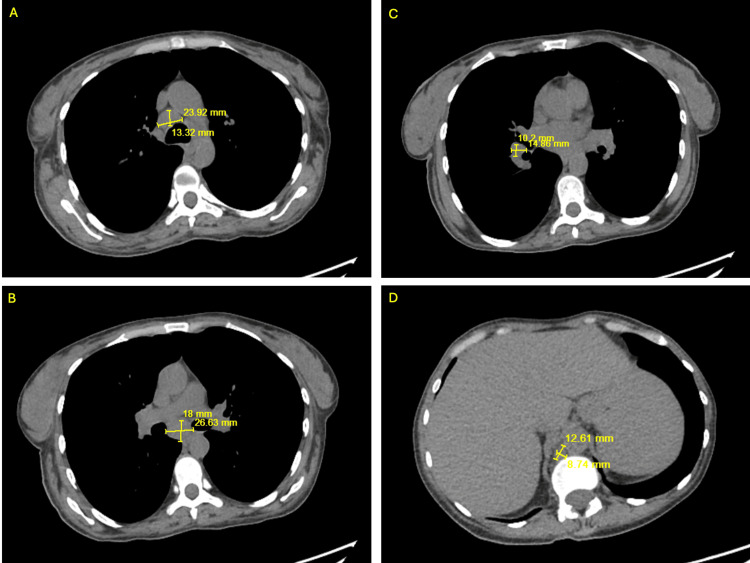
Computed tomography imaging of the chest demonstrating mediastinal and upper abdominal lymphadenopathy A-C: Axial view of multiple mediastinal lymph nodes. D: Axial view of upper abdominal adenopathy.
